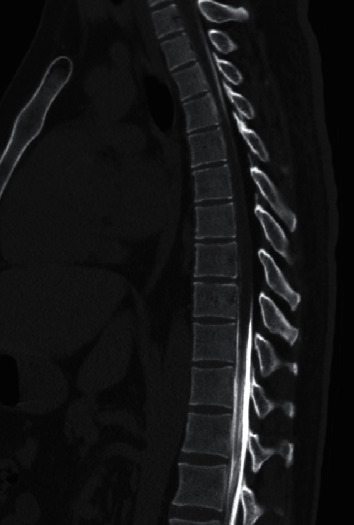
CT myelogram showing obstruction of CSF flow extending from T4 to T9 vertebrae.
